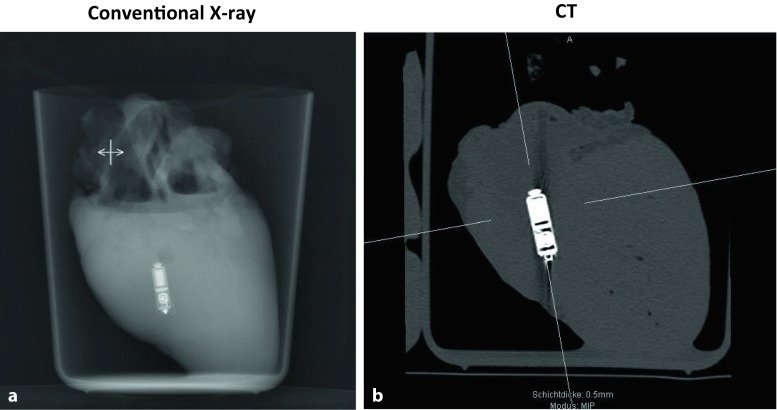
Visualization of the Micra pacemaker system in X‑ray (a) and in computed tomography (CT; b)
